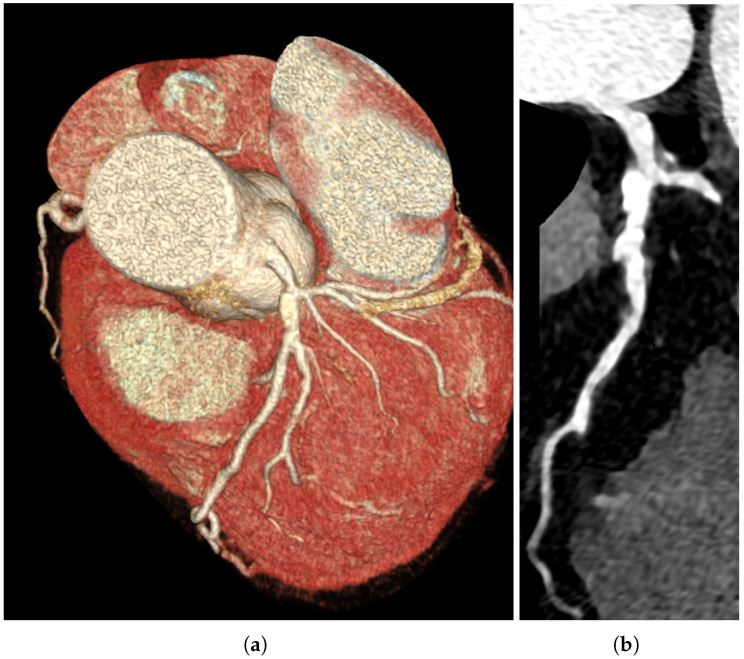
(a,b) Computed tomography angiography with borderline left main coronary artery stenosis with estimated minimal lumen area of 7 mm^2^.
